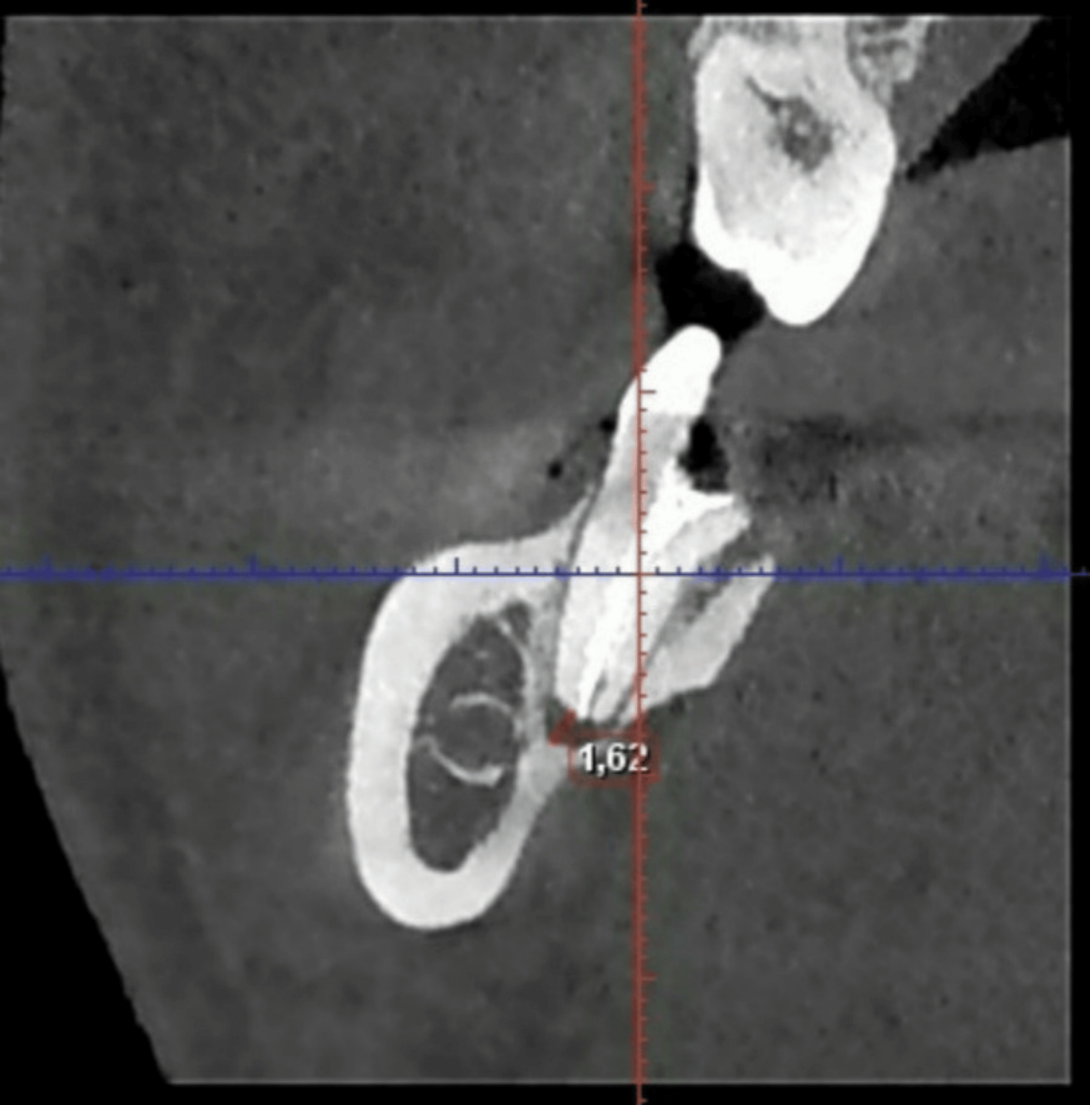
CBCT image of the distal root of tooth 47 showing measurements of the apical defect in coronal section. CBCT, cone beam computed tomography.
